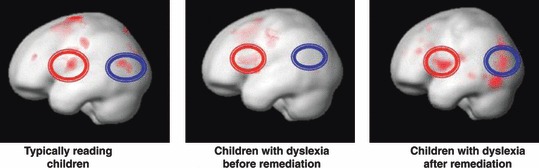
Brain activation differences in dyslexia and its treatment, based on data from Temple et al. (2003). Figure and explanatory legend (below) reproduced with permission from Gabrieli, J. D. (2009). Dyslexia: a new synergy between education and cognitive neuroscience. Science, 325 (5938), 280–283. ‘Functional magnetic resonance imaging activations shown on the left hemisphere for phonological processing in typically developing readers (left), age-matched dyslexic readers (middle), and the difference before and after remediation in the same dyslexic readers (right). Red circles identify the frontal region, and blue circles identify the temporo-parietal region of the brain. Both regions are hypoactivated in dyslexia and become more activated after remediation’
